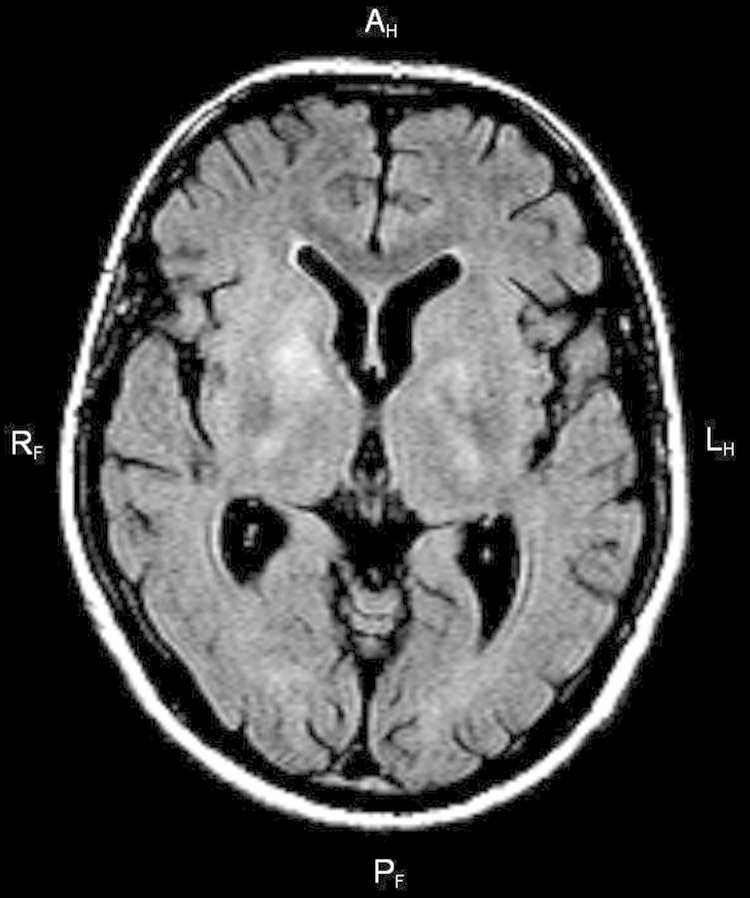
Fluid-attenuated inversion recovery magnetic resonance imaging of the brain of a
